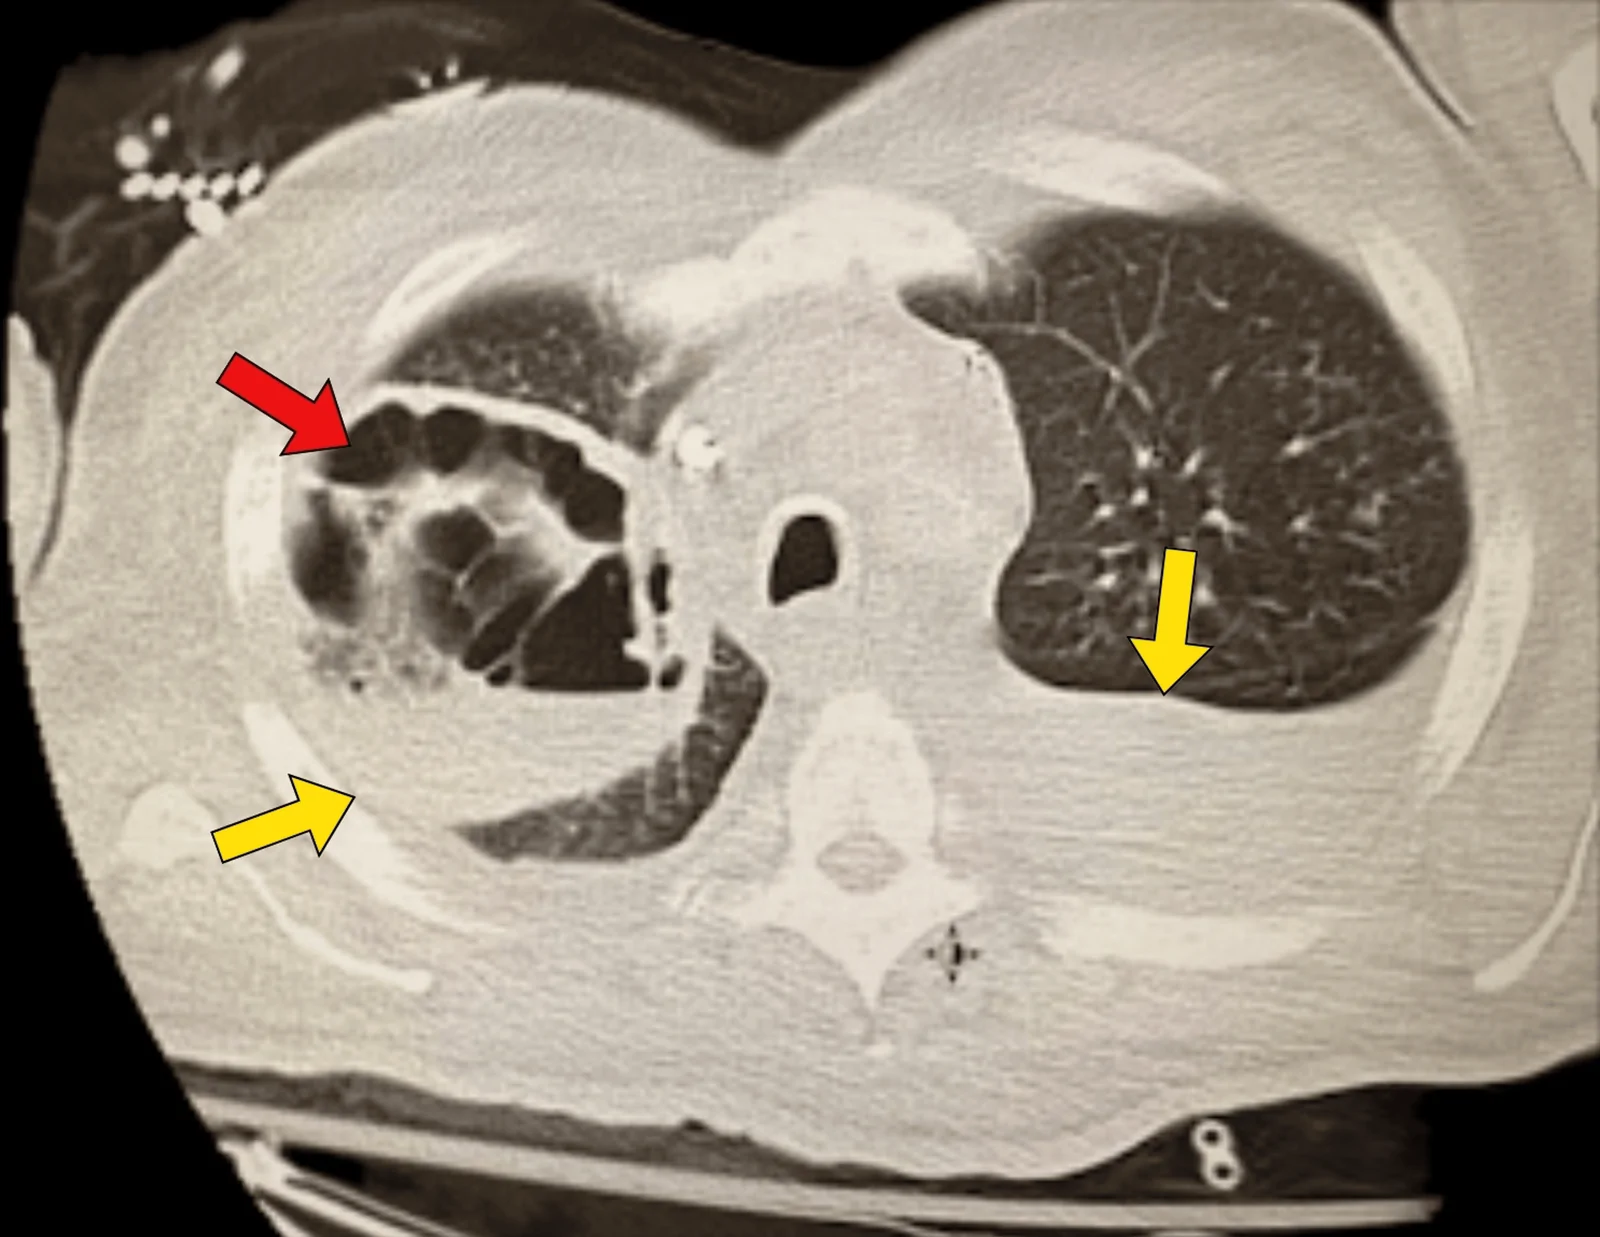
Axial CT image of the chest demonstrates the cavitary lesion (red arrow) and bilateral pleural effusions (yellow arrows). The combination of cavitary disease and pleural effusions prompted further evaluation for an additional infectious or malignant process.
